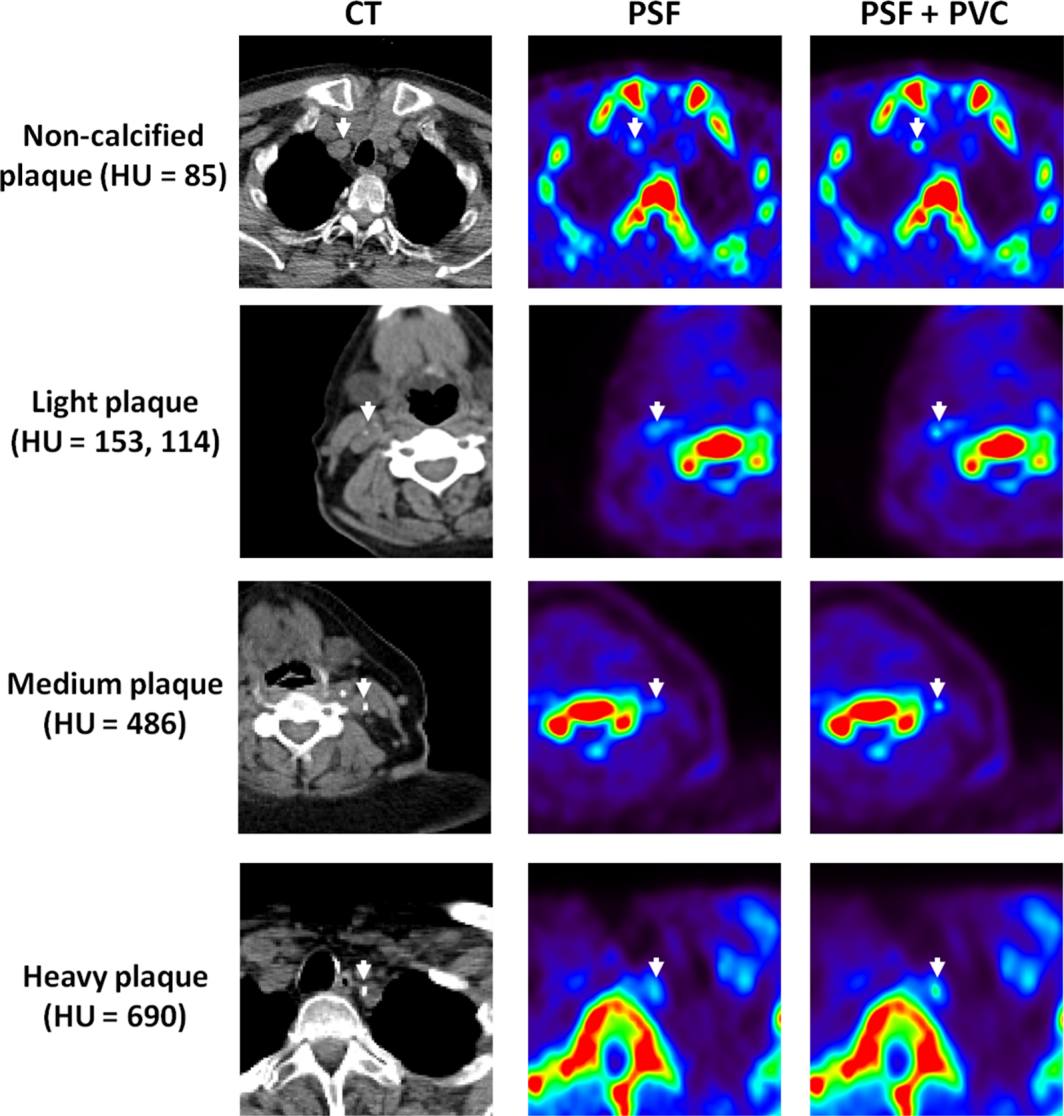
From *left* to *right*: CT, standard OSEM and PVC reconstructions of patients with atherosclerotic plaque in the carotids (*arrows* in CT images). From *top* to *bottom* we show images of patients with non-calcified plaque (HU < 110), light plaque (110 < HU < 210), medium (210 < HU < 550), and heavy plaque (HU > 550) accumulation
